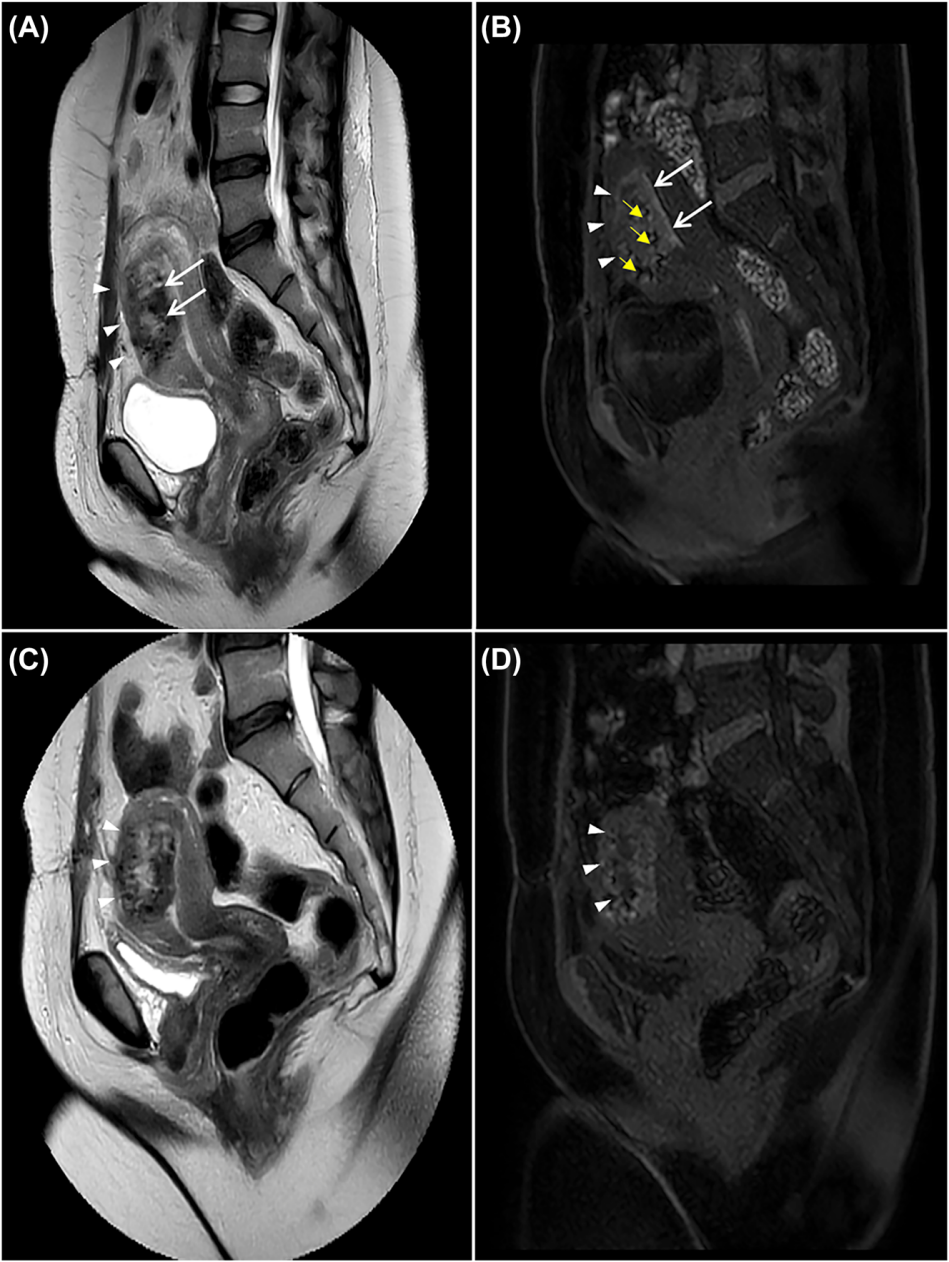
Sagittal precontrast (A) and subtracted post-contrast (B) T1-weighted images obtained on post-operative day 20. The images show completed absence of enhancement in the affected anterior myometrium. Note normal enhancement of the rest of the uterus. Due to characteristic appearances, these findings were consistent with a contained intramyometrial hematoma, related to Couvelaire uterus or post-surgical changes from classical cesarean delivery. Repeat MRI imaging two weeks after the initial study demonstrates interval evolution of the anterior intramyometrial hematoma. Sagittal (C, D) T2 and pre-contrast T1 weighted images demonstrate that the area is slightly smaller than the previous study, has increased T2 signal within its central aspect (C white arrow heads), possibly indicating interval liquefaction, and has a more prominent hemosiderin ring with reduced prominence of the precontrast T1 hyperintensity (D white arrow heads), especially along the anterior junctional zone. Findings are again consistent with diagnosis of intramural hematoma in the setting of the clinical diagnosis of Couvelaire uterus. There was no evidence of myometrial necrosis or abscess formation.
